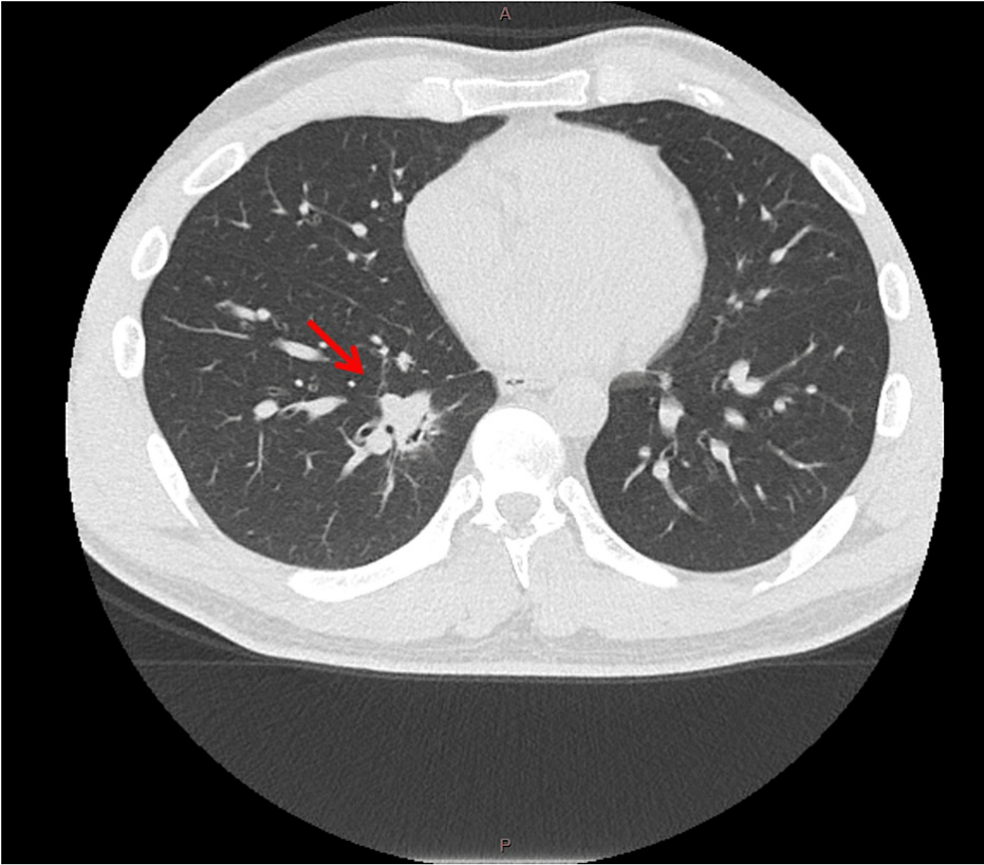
Chest CT image showing a right lower lobe nodule - arising adjacent to the right posterior basal segmental bronchus along with adjacent ground-glass airspace opacities likely reflecting pulmonary hemorrhage
